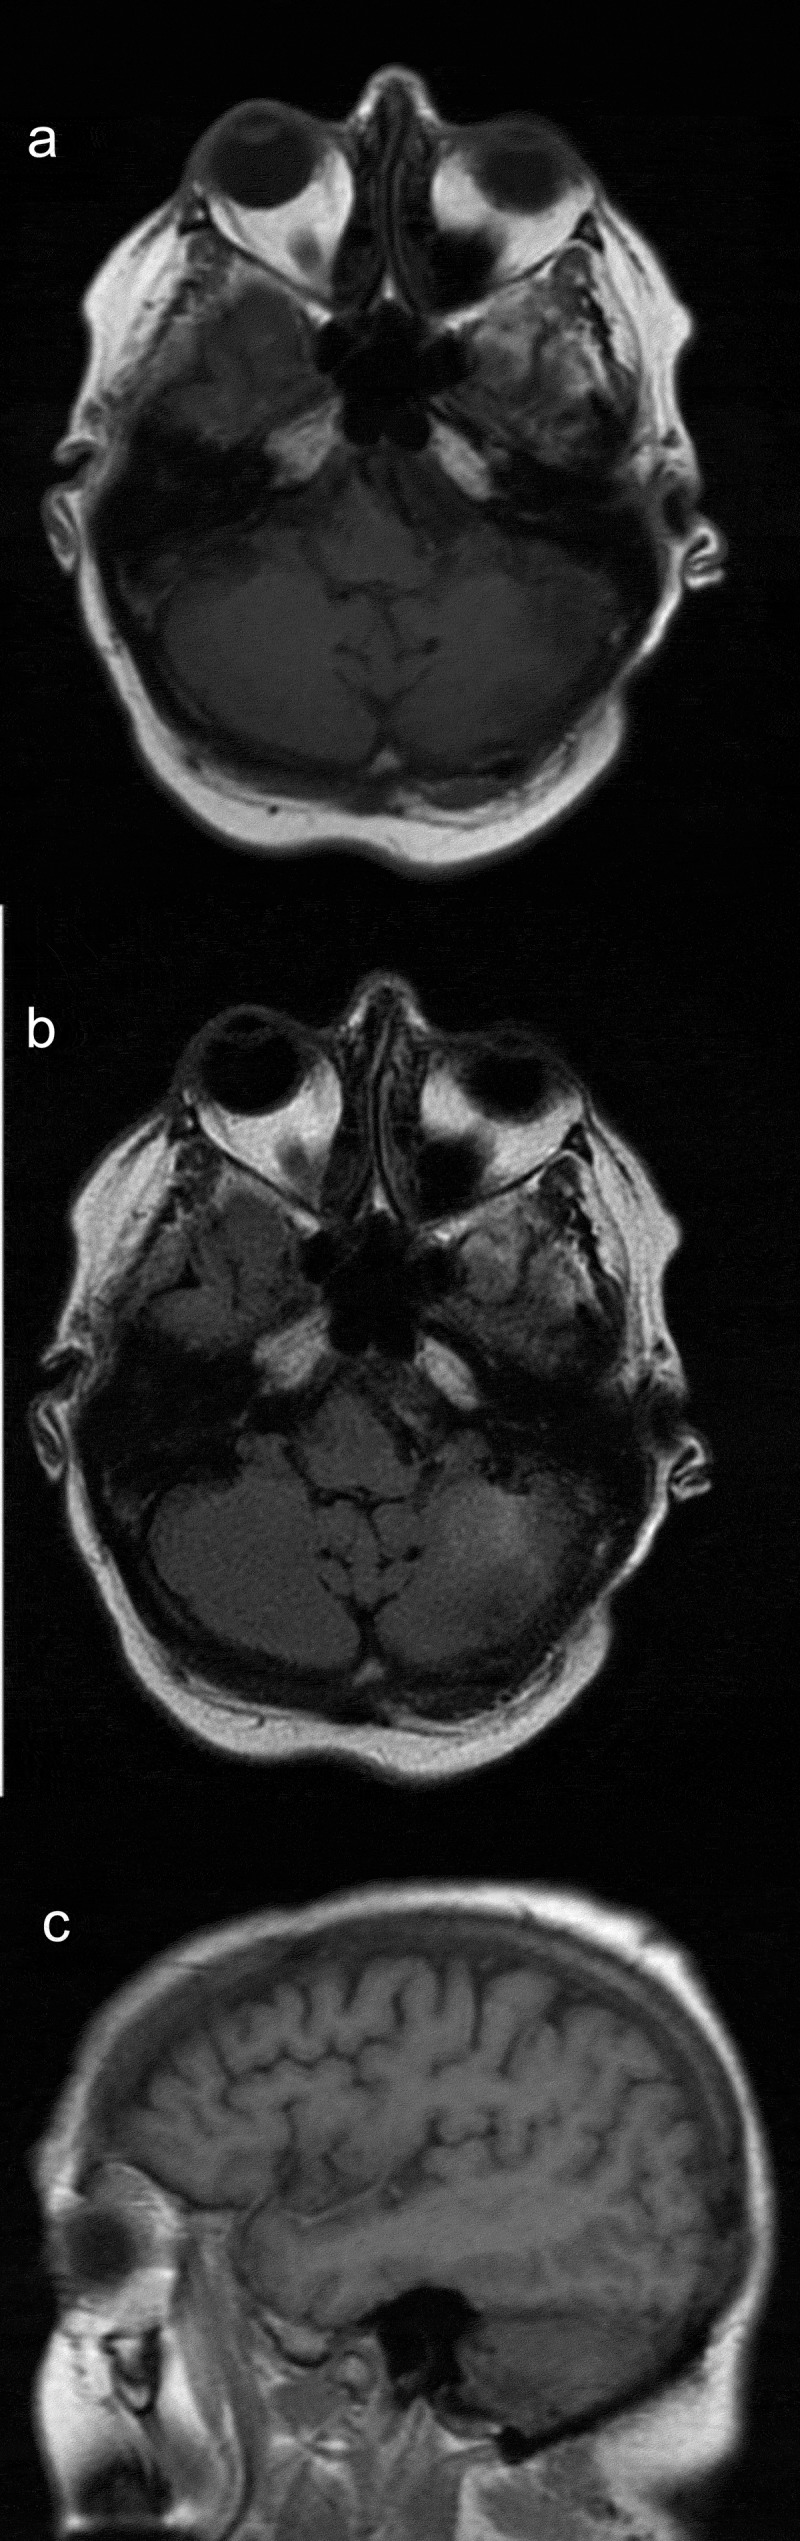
Follow-up MRI brain at 1 year showing evidence of prior resection of the left cerebellar mass with no recurrence of metastatic lesion or new metastasis.
